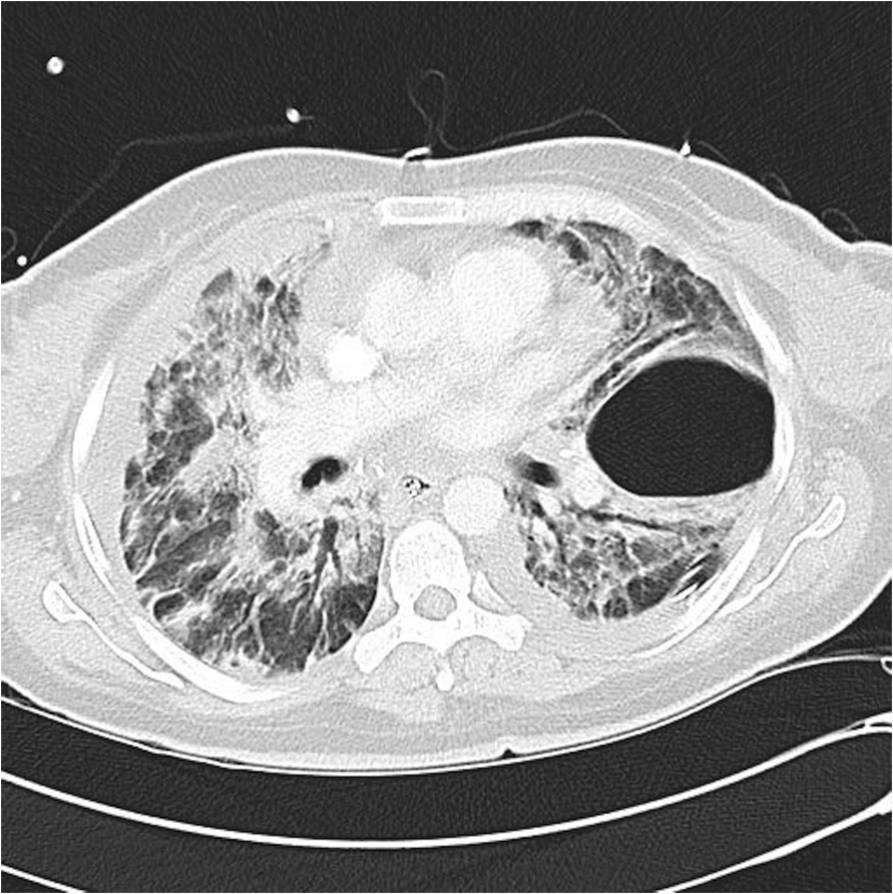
High resolution computed tomography scan of the chest. Extensive areas of air-space consolidations in both lungs and bilateral alveolar opacities. Pneumothorax at the level of the left lower lobe with bilateral pleural effusions.
